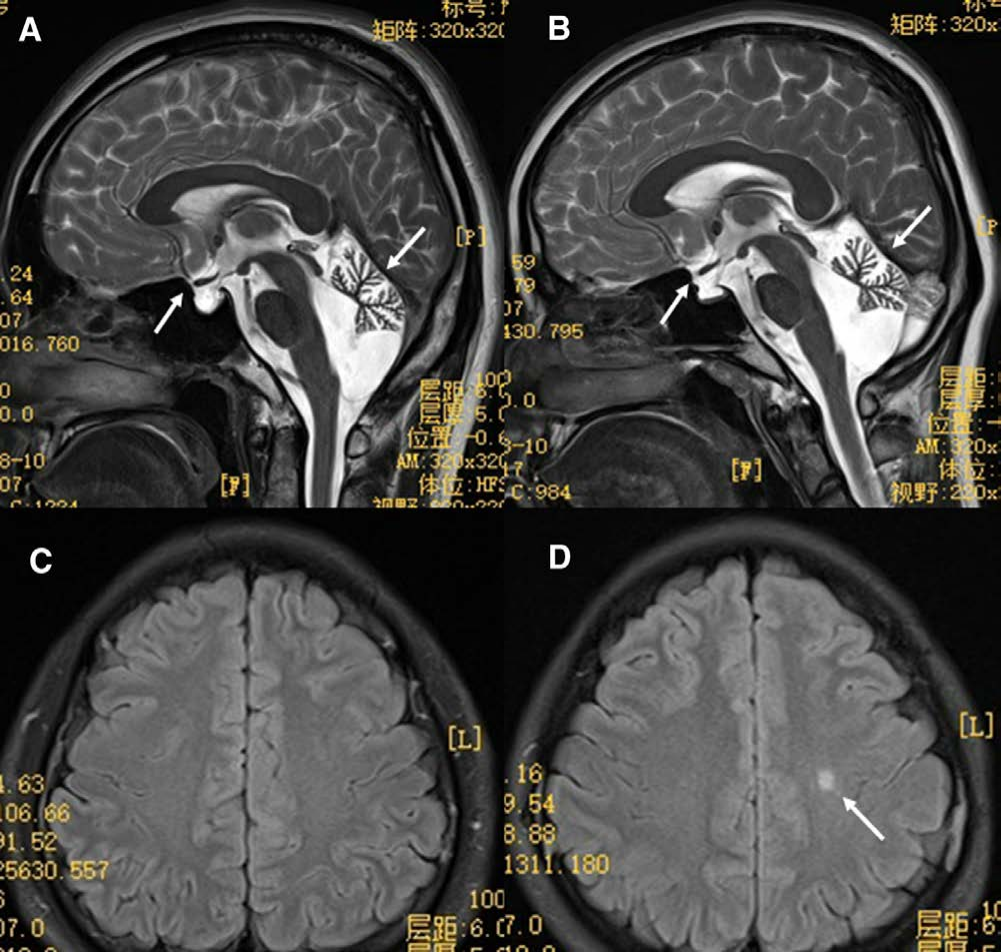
Brain MRI. (A) The cerebellar atrophy and hypophysis dysplasia from brother and (B) from sister. (C) Does not have obvious demyelination in brother. (D) The local demyelination in sister. MRI = magnetic resonance imaging.
